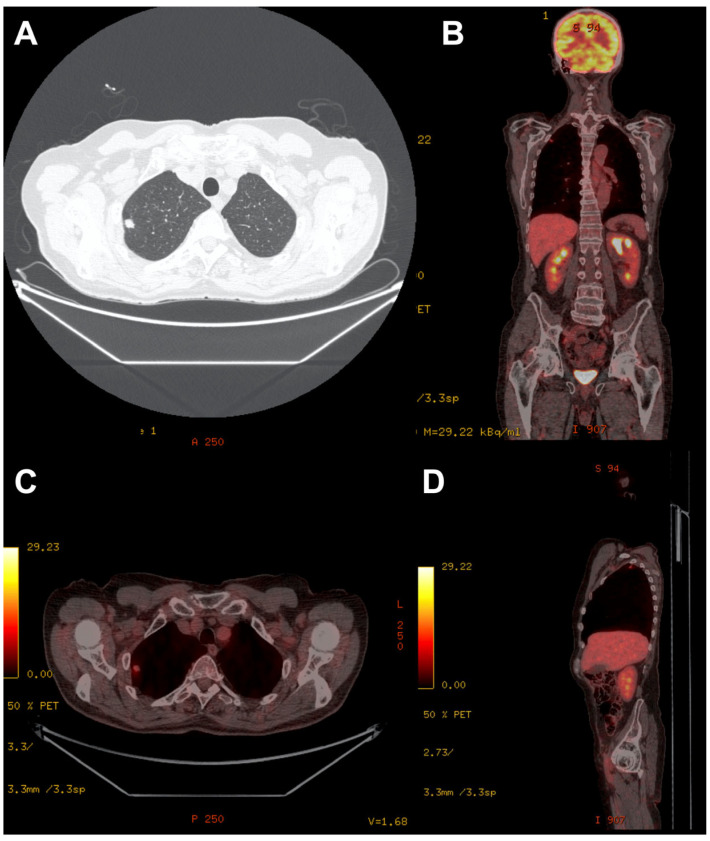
(A) Chest CT with a nodule in the right lung. (B). PET-CT coronal view. (C). PET-CT axial view. (D). PET-CT sagittal view. An 11 mm nodule is present in the second segment of the right lung, with a standardized uptake value of 2.45.
